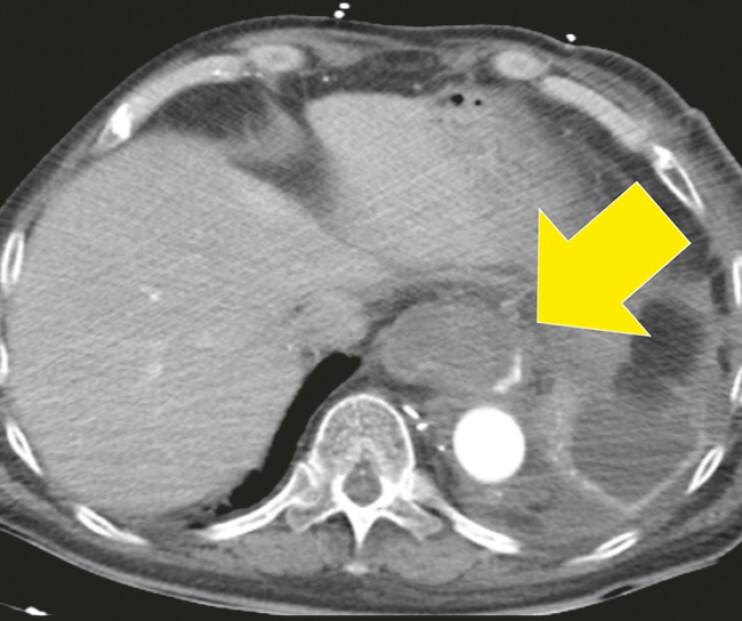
Contrast-enhanced computed tomography (CT) revealed an epiphrenic esophageal diverticulum on the left side of the distal esophagus, with active extravasation from the site (arrow).
